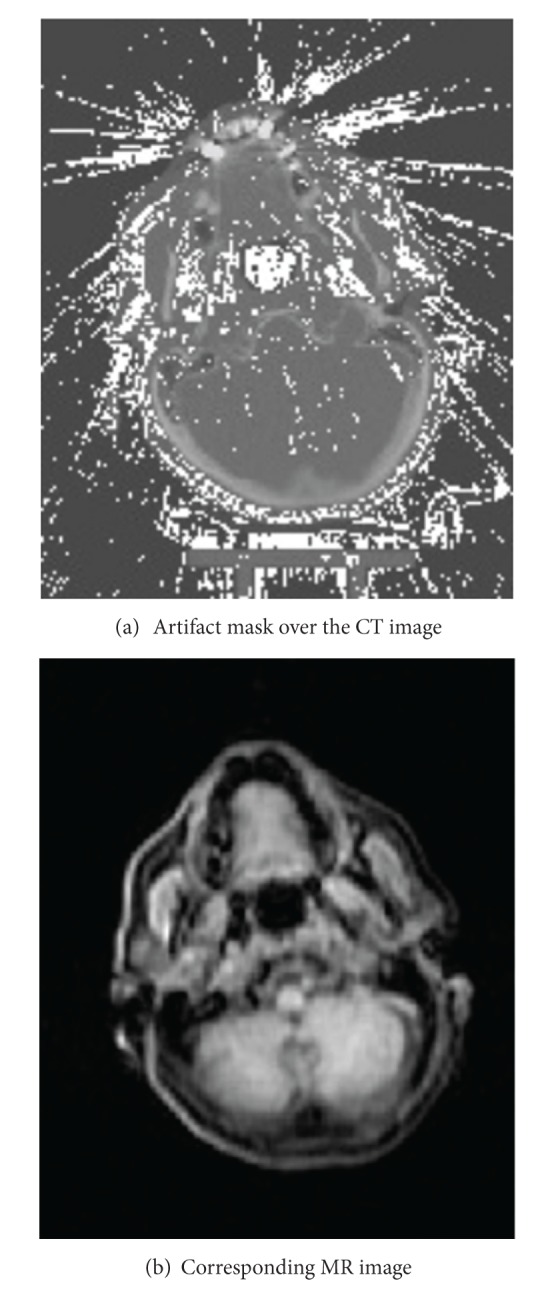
Detected artifacts on CT image (a) and corresponding MR image (b).
